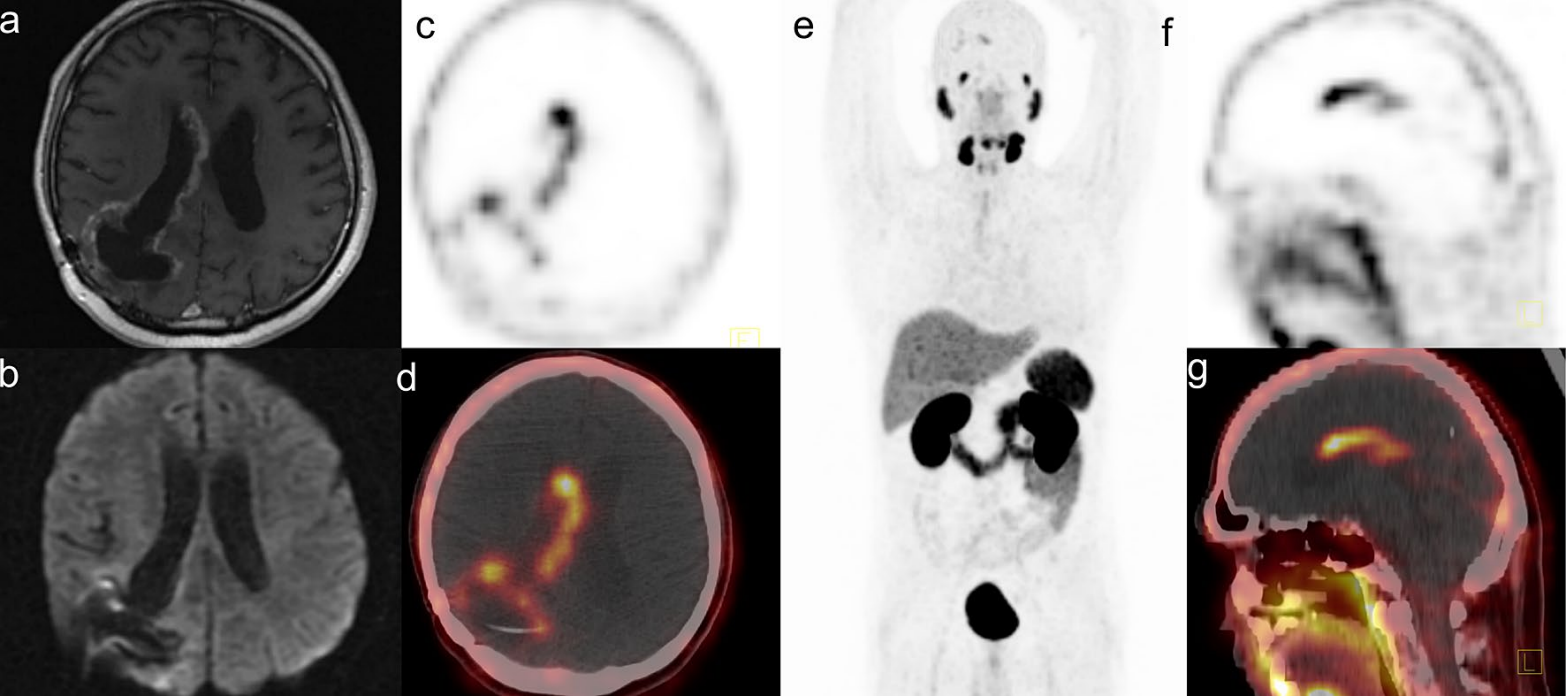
Female, 42 years, with a recurrent secondary glioblastoma in the right parietal lobe. Initially, she was diagnosed with glioma tumor grade II and underwent surgery. A follow-up MR revealed a contrast-enhanced mass around the surgical cavity 119.2 months after primary treatment. PET/CT showed high [^68^Ga]Ga-PSMA-11 accumulation in the tumor; with the SUV_max_ 17.4, SUV_mean_ 7.4, TBR 870 and TLR 3.4 (a—contrast-enhanced T1WI, b—DWI, c—PET transverse image, d—fused PET/CT transverse image, e—maximal intensity projection, f—PET sagittal image, g—fused PET/CT sagittal image).
